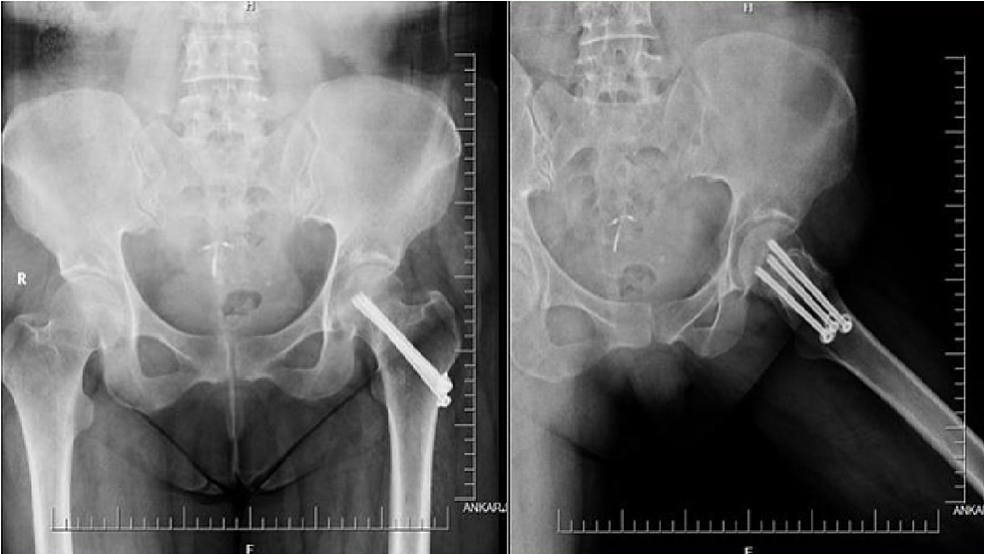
Postoperative anteroposterior and lateral radiographs of a 43-year-old female patient
